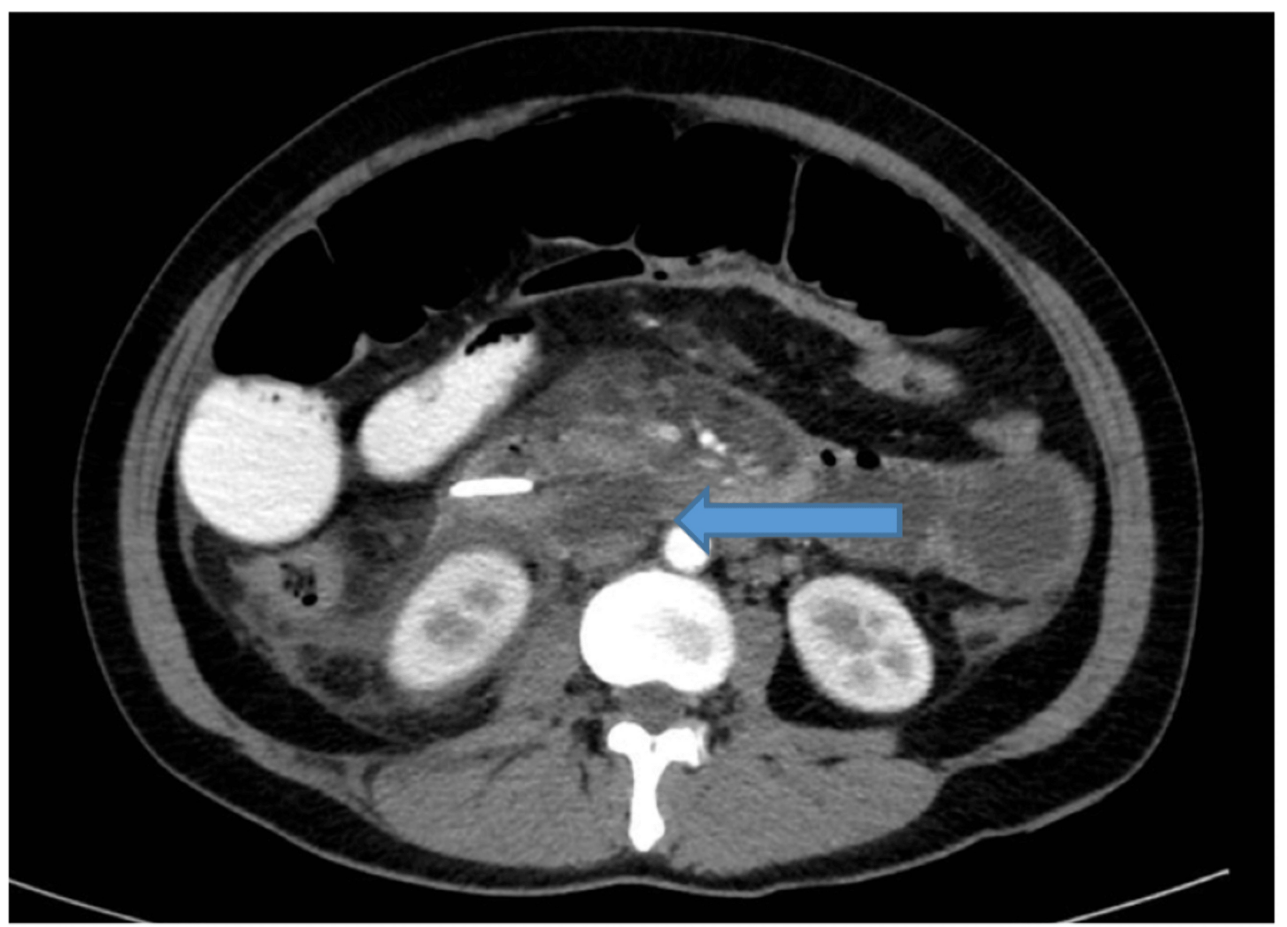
CT scan showed a 15 × 37 mm retroperitoneal fluid and gas collection posterior to the D2-D3 segments of the duodenum.
